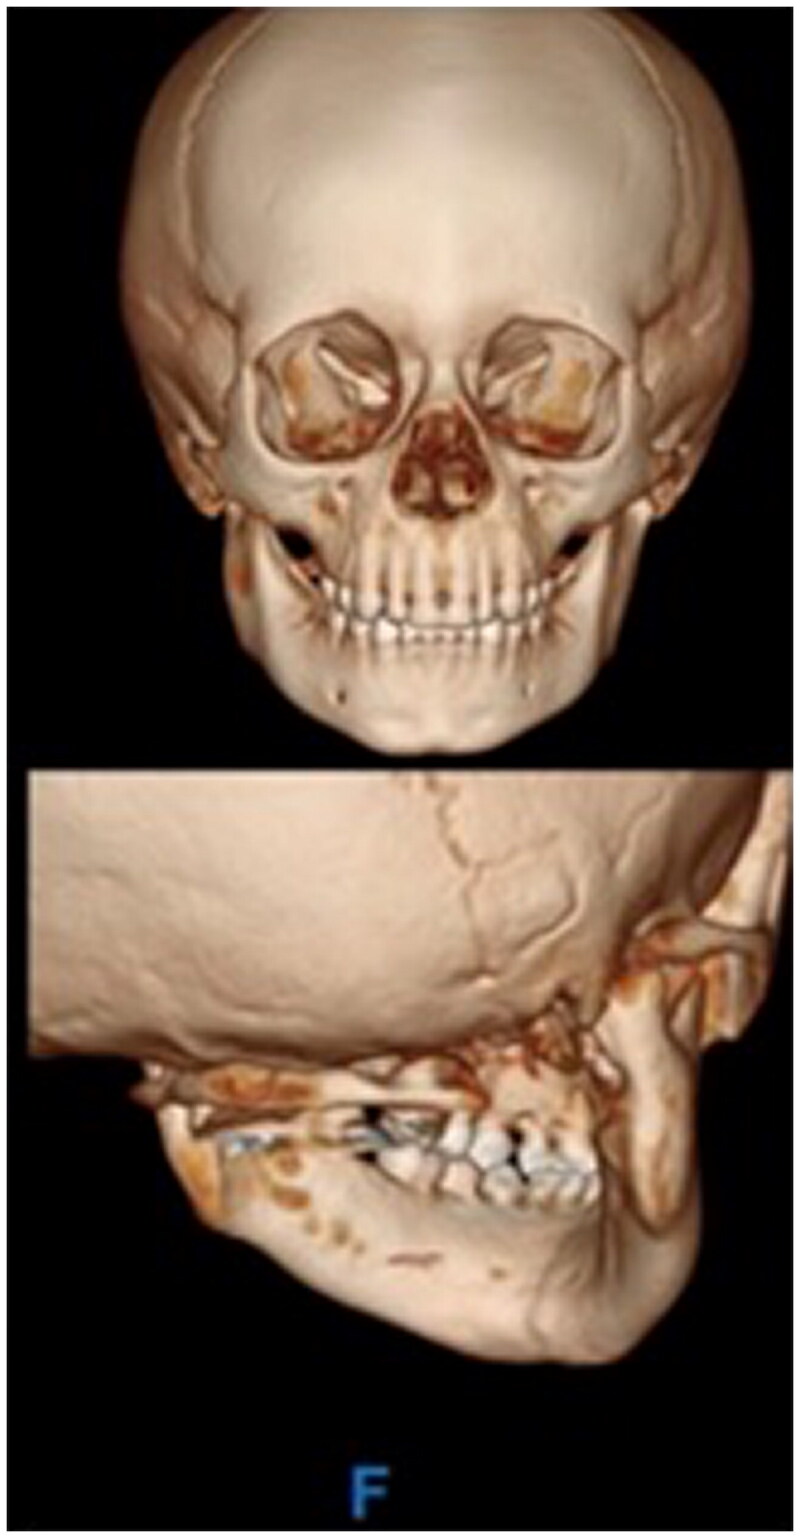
The follow-up CT maxillofacial scan at 5-months post-initial surgery demonstrated relocation, remodeling, and bony healing. Specifically, the left TMJ is restored and relocated, the right condylar fracture has remodeled and is in nearly anatomic pre-injury location, and the symphyseal fracture has healed.
